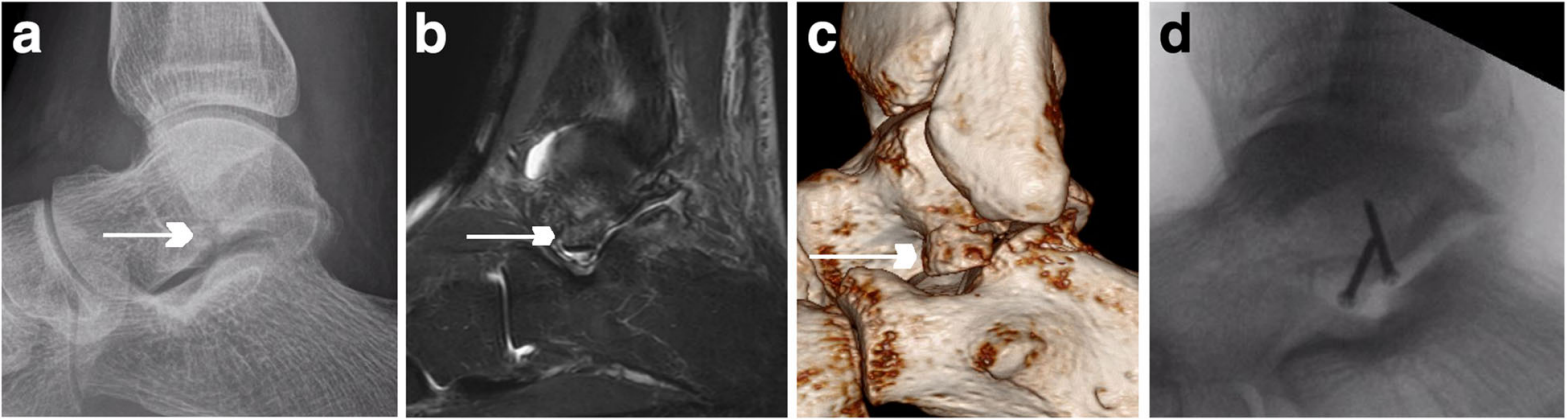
Clinical example of a patient with a lateral talar process fracture (LTPF). a Conventional lateral radiograph. b MRI showing a sagittal proton density fat-saturated image, which demonstrates the fracture line (*arrow*). c Computed tomography images confirming the displaced lateral talar process fracture (*arrows*). d Conventional lateral radiograph after open reduction and internal fixation with two screws
